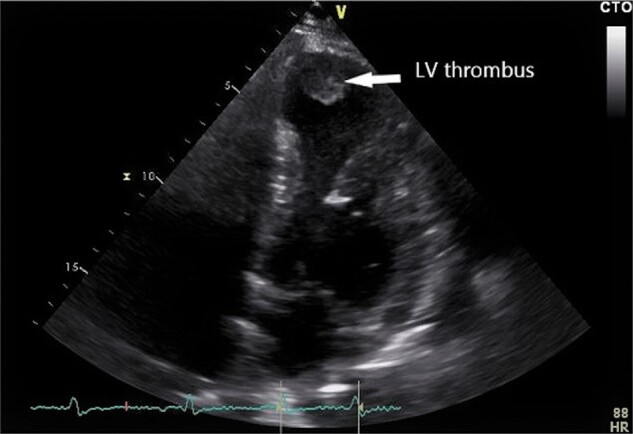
Transthoracic echocardiography. Four-chamber view showing the thrombus in the left ventricle.
